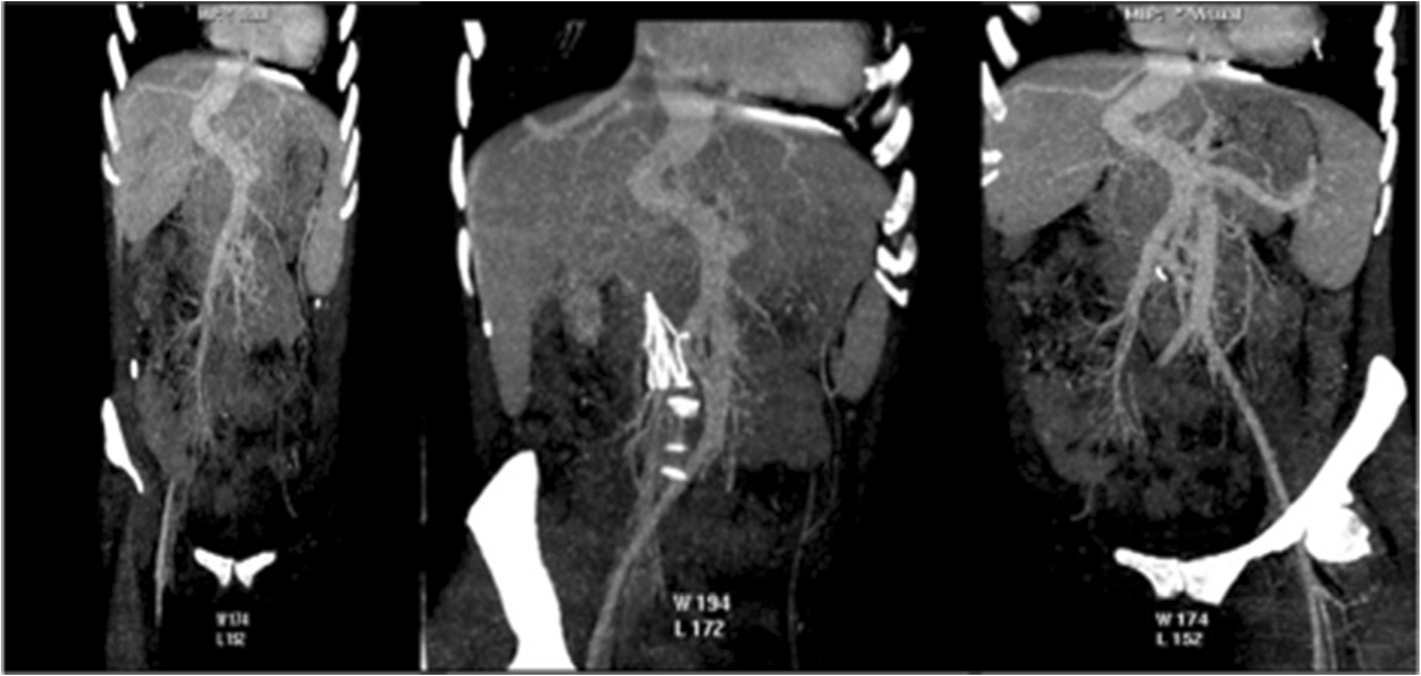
CT coronal planes in arterial and portal phases on case 1 (Type 1 – end-to-end). Note: Direct portal systemic shunt with evidence of the longitudinal path along the upper mesenteric vein, portal vein axis with the deviation and a kinking route could be observed. Without any hepatic vein intra and finishing in inferior cava vein near the right atrium
